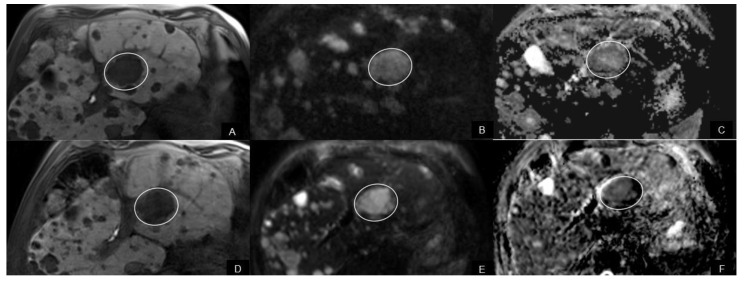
A 66-year-old male with liver metastases of pNET classified as a responder. (A) The pre-interventional axial T1w image (hepatobiliary phase) shows hypointense metastasis (circle) in segment 2. The metastasis (arrow) shows restricted diffusion with high signal on axial DW-MR image b = 800 s/mm^2^ (B,D) dark signal (circle) on ADC map (C). The pretherapeutic ADCmin value of the metastasis was 858 × 10^−3^ mm^2^/s. Under everolimus, the metastasis showed no significant change in size on hepatobiliary phase (D), however there was a loss of signal on DW-MR image b = 800 s/mm^2^ (E) compared to the pre-interventional image and decreasing signal (circle) on the ADC map (F). The post-interventional ADCmin values were 476 × 10^−3^ mm^2^/s and lower higher compared to pretherapeutic ADC value.
